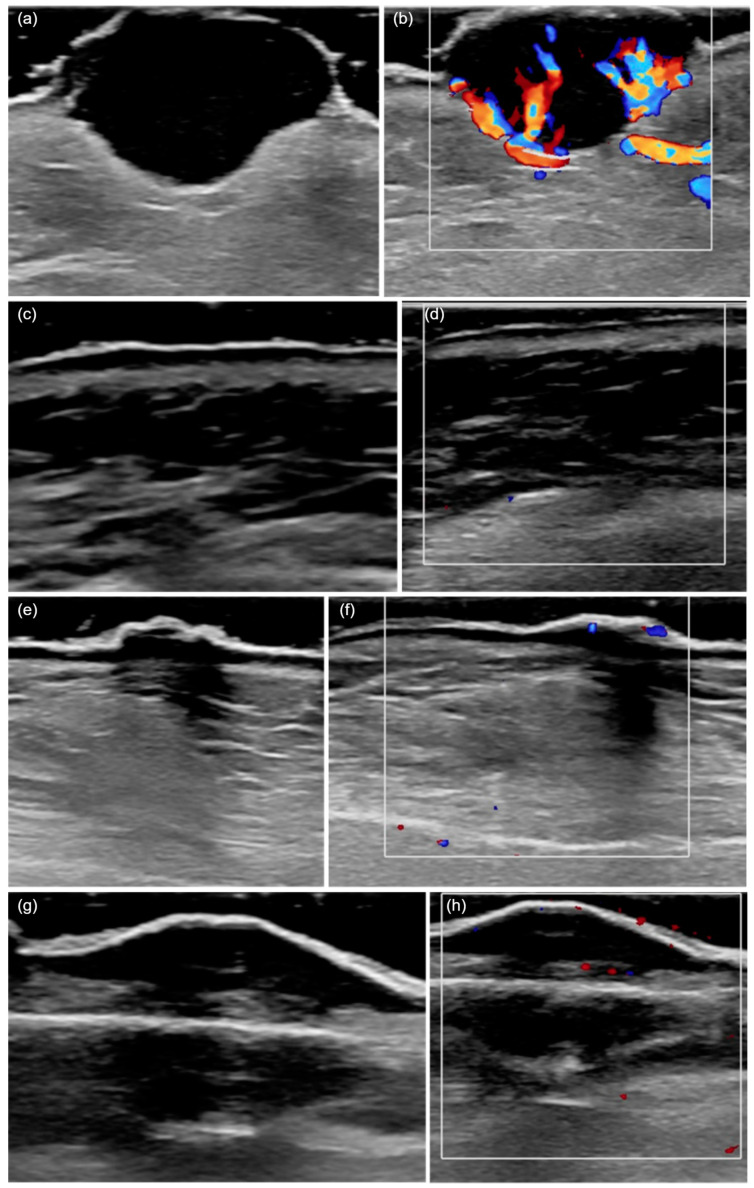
HFUS (20 MHz) features of cutaneous squamous cell carcinoma. (a) A hypoechoic lesion with an irregular shape and heterogeneous echogenicity, invading the deep dermis and presenting ulceration. (b) The color Doppler mode shows a marked increase in local vascularization. (c) A hypoechoic tumor confined to the epidermis, with a regular shape and homogeneous echogenicity. (d) An absence of local vascularization in color Doppler mode. (e) The presence of posterior acoustic shadowing. (f) Moderate vascularization detected in color Doppler mode. (g) A hypoechoic, regular, and poorly defined lesion invading the hypodermis, with posterior acoustic shadowing. (h) The presence of vascularization in color Doppler mode.
